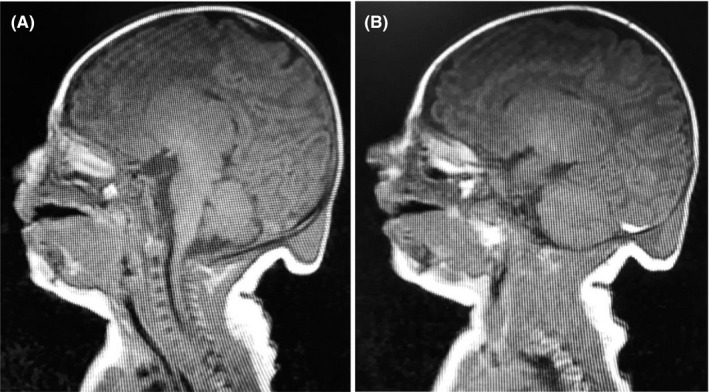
Sagittal magnetic resonance images showing (A) a small hyperintense collection in the left median‐paramedian occipital site, located under the skull; and (B) a fluid oval collection in the retronuchal site, with an inhomogeneous signal and a small fluid level in its context
